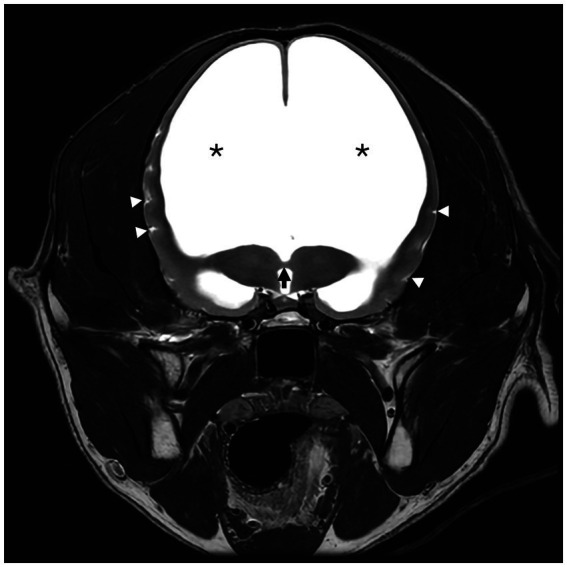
T2-weighted (T2W) transverse magnetic resonance image (MRI) of a 6-year-old male neutered golden retriever. The interthalamic adhesion is markedly thinned (black arrow). The septum pellucidum and corpus callosum are absent. Marked lateral ventriculomegaly is denoted by (*). There is decreased CSF signal within the sulci of the remaining thin cerebral cortex (white triangles).
